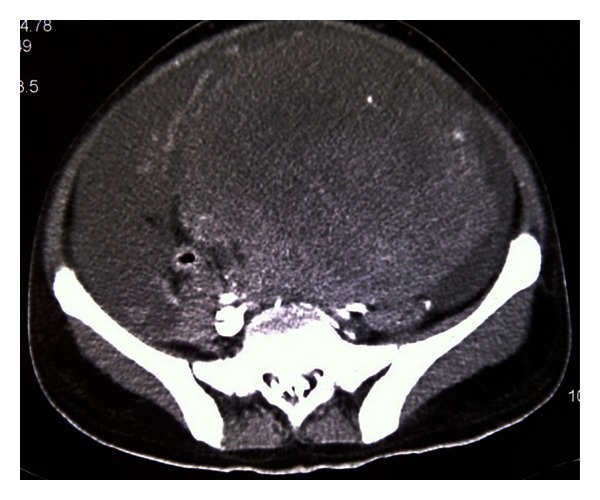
CT abdomen at presentation showed huge mass.
